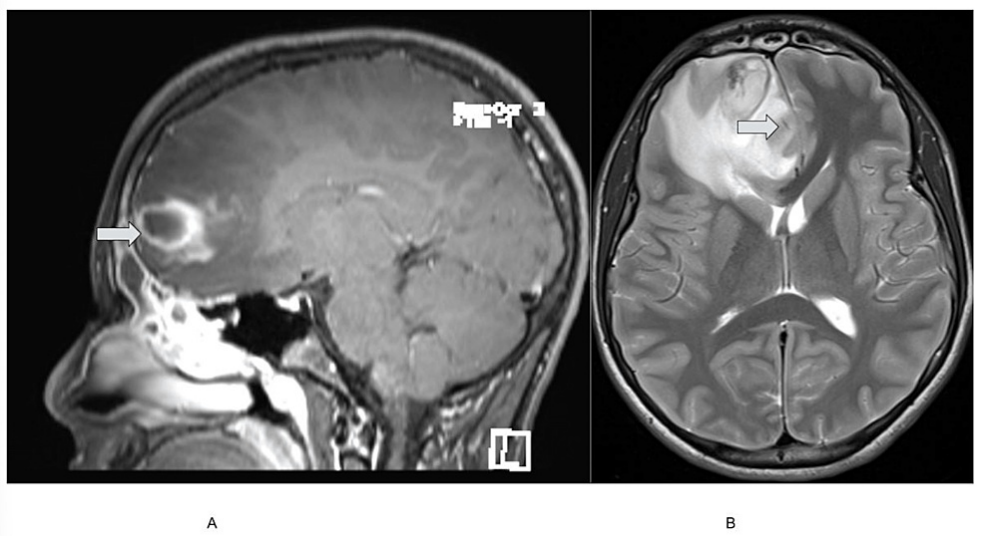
Admission (symptom day 21) magnetic resonance imaging of the brain with contrast. (A) 2.5 cm rim-enhancing fluid collection in the right frontal lobe. (B) 10 mm of anterior midline shift.
